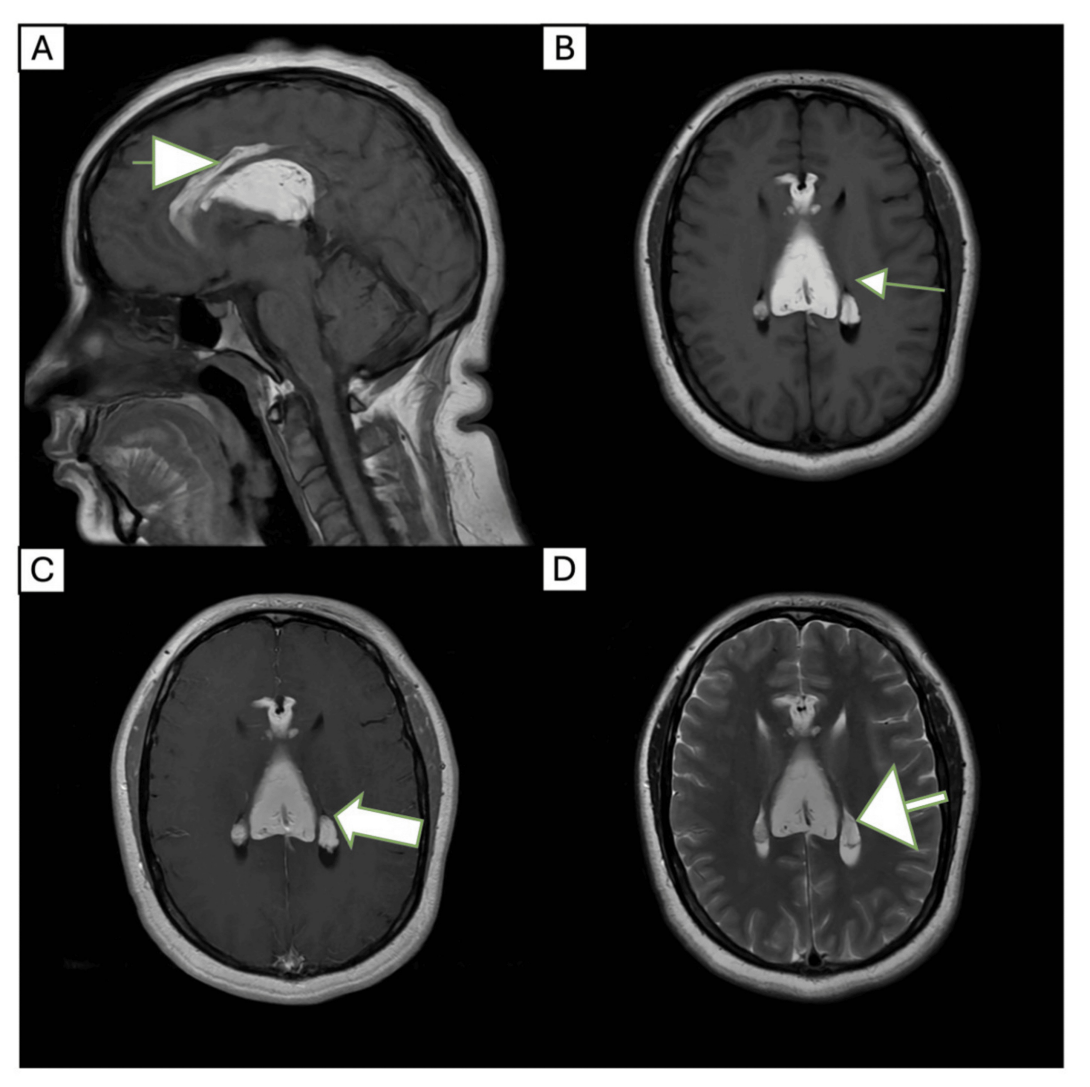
MRI brain with and without contrast was performed in 2024. Sagittal T1-weighted image (A), axial T1-weighted image (B), axial T1 post-contrast (C), and axial T2-weighted image (D) demonstrate a well-circumscribed, non-enhancing midline fatty mass measuring 6.1 × 3.3 cm. The lesion is located along the corpus callosum and is associated with callosal dysgenesis, consistent with a diagnosis of pericallosal lipoma. MRI: magnetic resonance imaging
